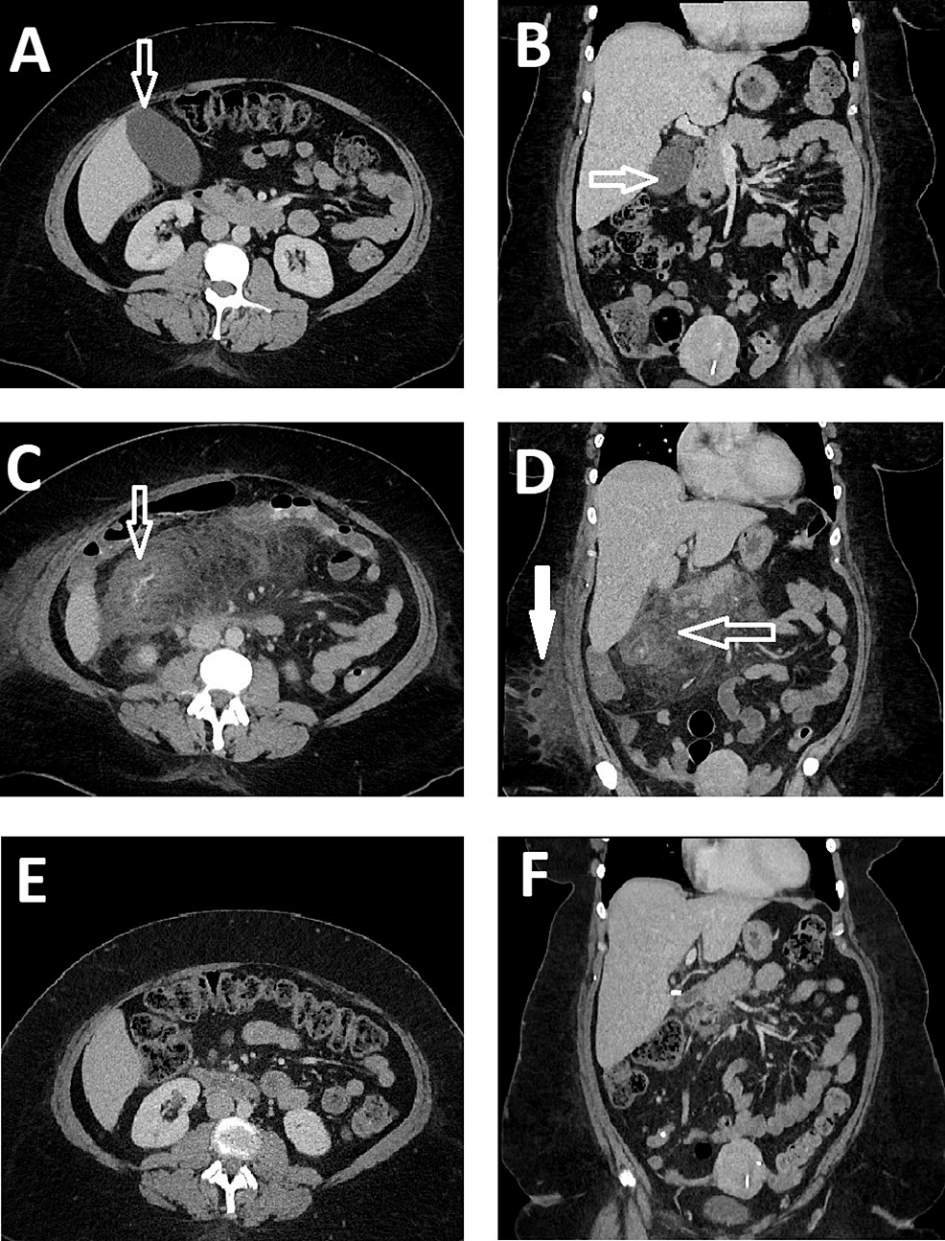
Preoperative and postoperative CT abdomen and pelvis demonstrating postoperative localized colitis A) Representative axial image of the preoperative CT scan of the abdomen and pelvis with a hollow arrow pointing at a distended gallbladder; B) Representative coronal image of the preoperative CT scan of the abdomen and pelvis with a hollow arrow pointing at a distended gallbladder; C) Representative axial image of the CT scan of the abdomen and pelvis on postoperative day four with a hollow arrow pointing at severe inflammatory changes of the hepatic flexure at the proximal third of the transverse colon with stranding of the dependent visceral and retroperitoneal fatty tissue; D) Representative coronal image of the CT scan of the abdomen and pelvis on postoperative day four with a hollow arrow pointing at severe inflammatory changes of the hepatic flexure at the proximal third of the transverse colon with stranding of the dependent visceral and retroperitoneal fatty tissue. The solid arrow is pointing at the stranding of the peripheral fatty tissue; E) Representative axial image, and F) Representative coronal image of the CT scan of the abdomen and pelvis one month postoperatively with complete resolution of the severe localized inflammatory changes of the hepatic flexure at the proximal third of the transverse colon and dependent fatty tissue.
